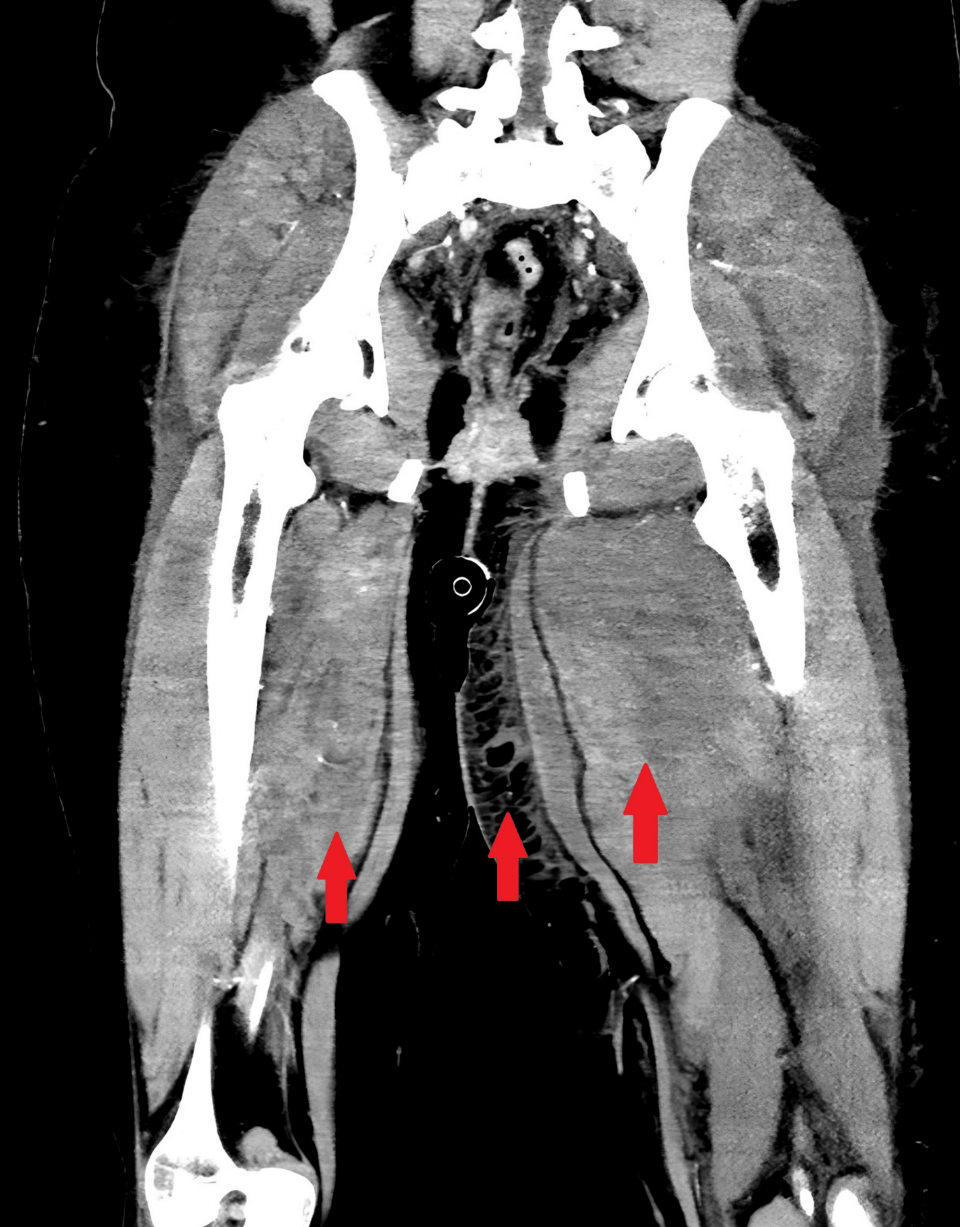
Coronal post-contrast CT of the proximal bilateral lower extremities Coronal contrast-enhanced CT shows bilateral, left greater than right, hip, and thigh muscle heterogeneous enhancement with muscle enlargement. Arrows mark areas of heterogenous enhancement and muscle enlargement bilaterally as well as inflammatory changes of the subcutaneous tissue of the thigh noted in the preceding figure.
